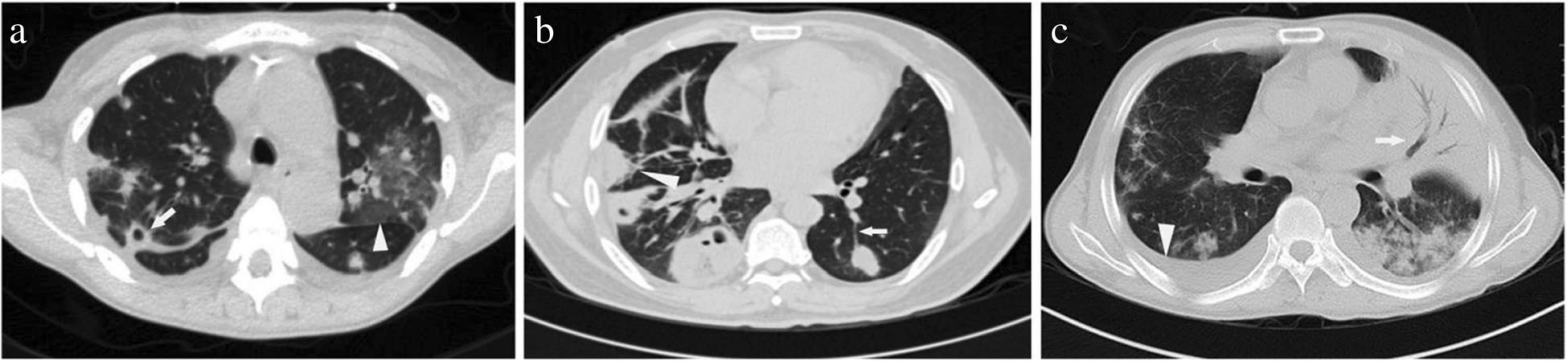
Chest CT revealed multiple peripheral nodules with cavitation (arrow, a) and ground-glass opacities (arrowhead, a); peripheral nodules with feeding vessel sign (arrow, b) and a wedge-shaped peripheral lesion (arrowhead, b), consolidation and air bronchogram (arrow, c) and pleural effusion (arrowhead, c)
